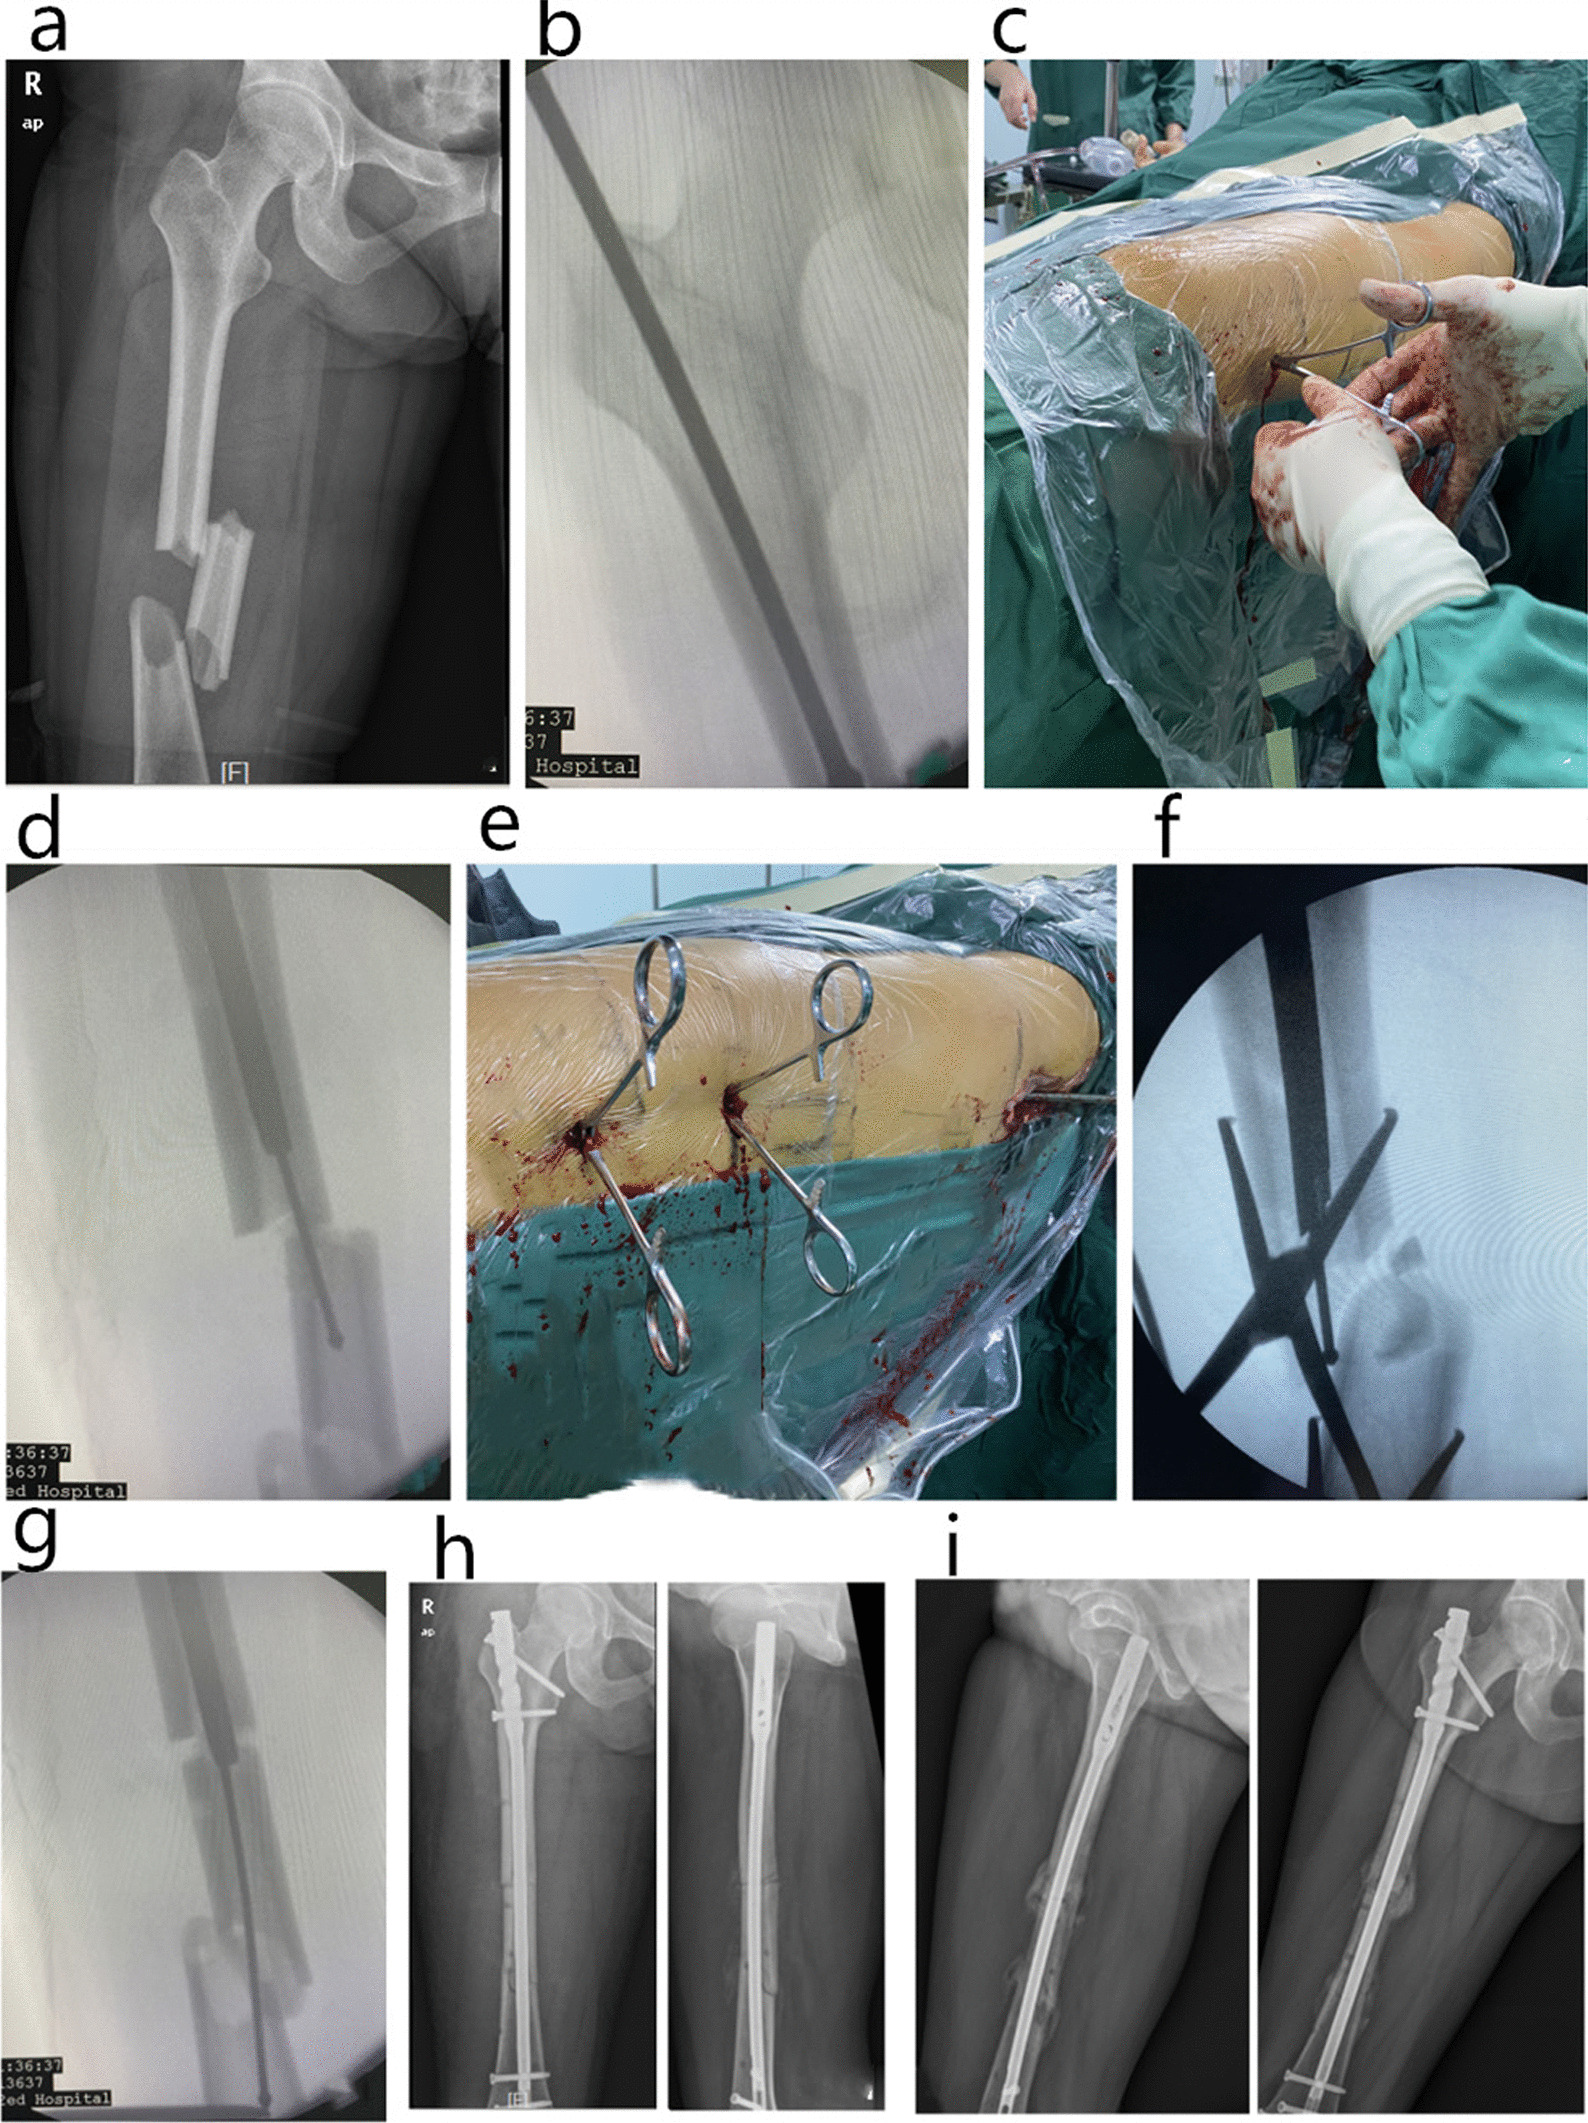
A 35-year-old female patient with a multi-segment femoral shaft fracture caused by a traffic accident. a Preoperative X-ray shows that the AO type of femoral shaft fracture was a 32-C fracture. b The intramedullary nailing point was ascertained and the guide wire was inserted; c An incision of 0.5 cm was cut in the skin by the lateral side of the medial femoral fracture block and toothed vascular forceps were inserted. d The intermediate fracture block was clamped using the toothed vascular forceps, and the guide wire was pushed by controlling the direction of the fracture block. e–g In the same way, the toothed vascular forceps were inserted into the lateral side of the fracture block at the distal end of the femur, and the guide wire was inserted, while the direction of the fracture block at the middle and the distal end was controlled. h An intramedullary nail after reaming. i Radiographs at 6 months postoperatively
